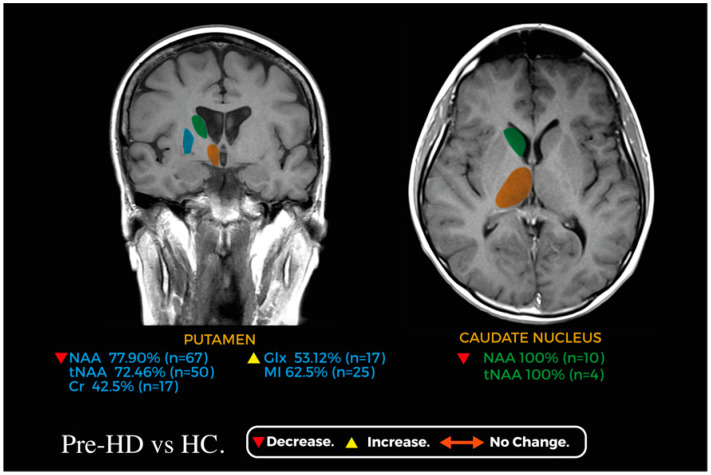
Coronal and axial planes of a magnetic resonance image representing the main changes in the metabolic profile of the caudate nucleus (green) and putamen (blue) between pre-HD and HC; the thalamus (orange) is shown for reference.
